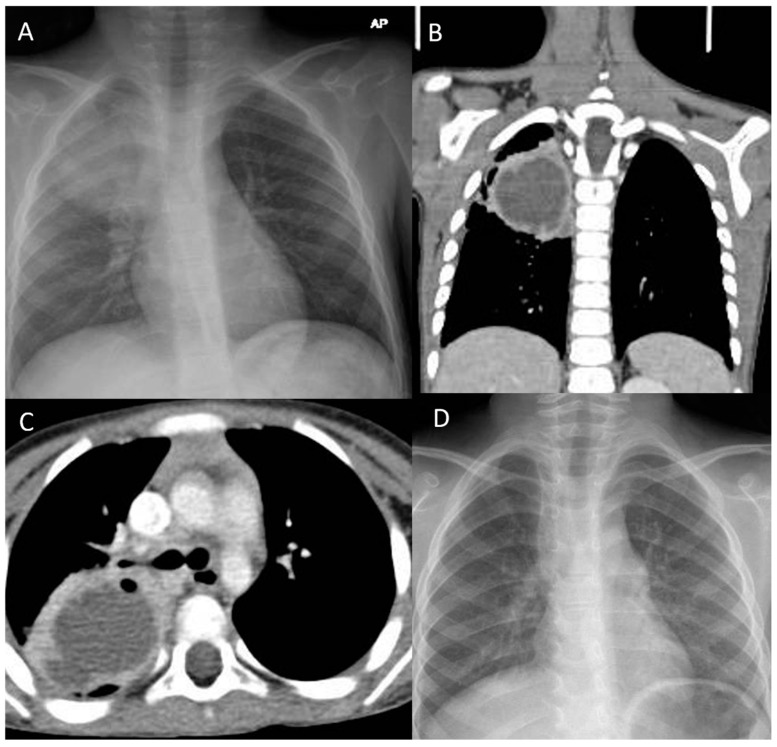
Chest X-ray (CXR) and chest computed tomography (CT) findings in case 5: (A) CXR of the patient on admission shows opacification involving the right upper lobe. (B,C) Coronal and axial windows, mediastinal view; CT scan of the chest shows a large, thick-walled cavity filled with fluids with heterogeneous opacity occupying the right upper lobe, leading to pleural and chest wall adhesions. (D) CXR of the patient at the 5-month postoperative follow-up shows well-aerated lung without any consolidations or air leakage.
